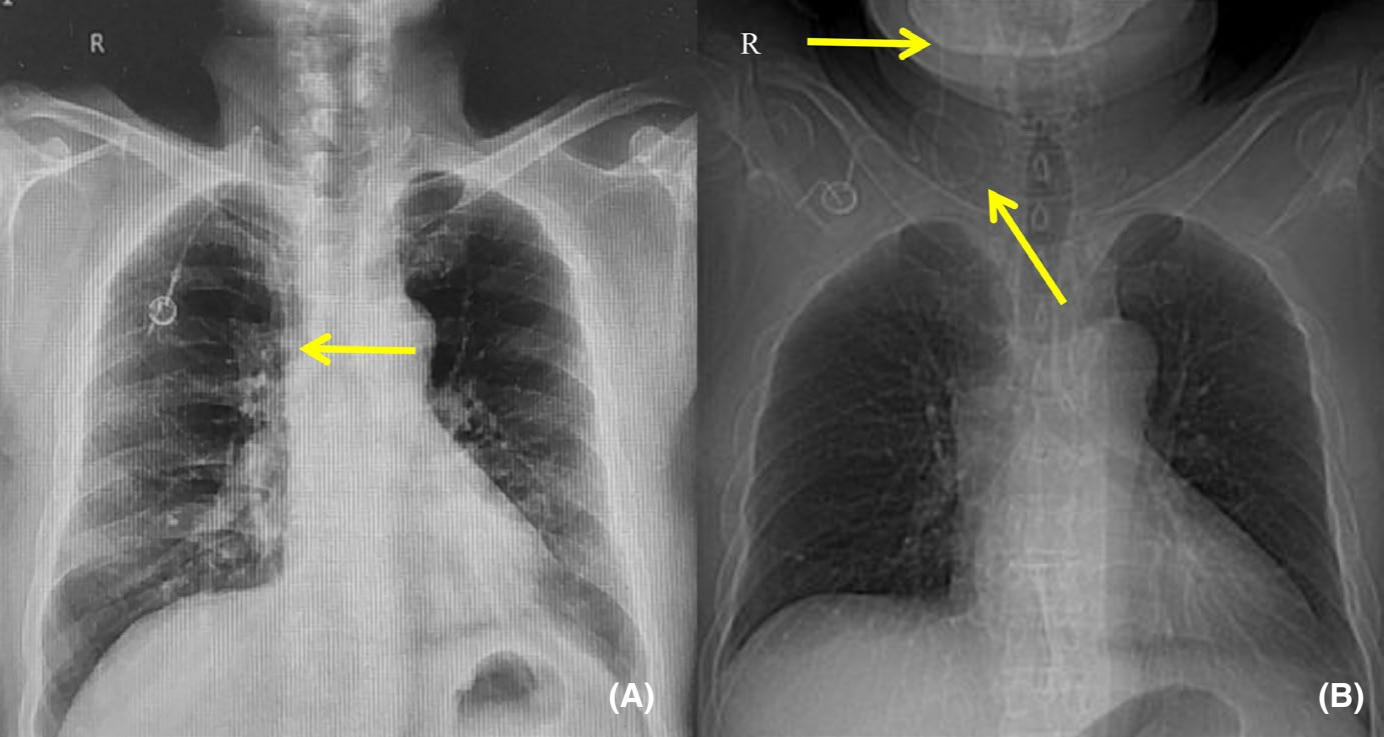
X‐ray radiography findings in Case 3. A, Postoperative X‐ray radiography showed that the catheter tip was correctly positioned. B, A second X‐ray examination revealed that the catheter tip had entered the lower craniocerebral segment of the right IJV
